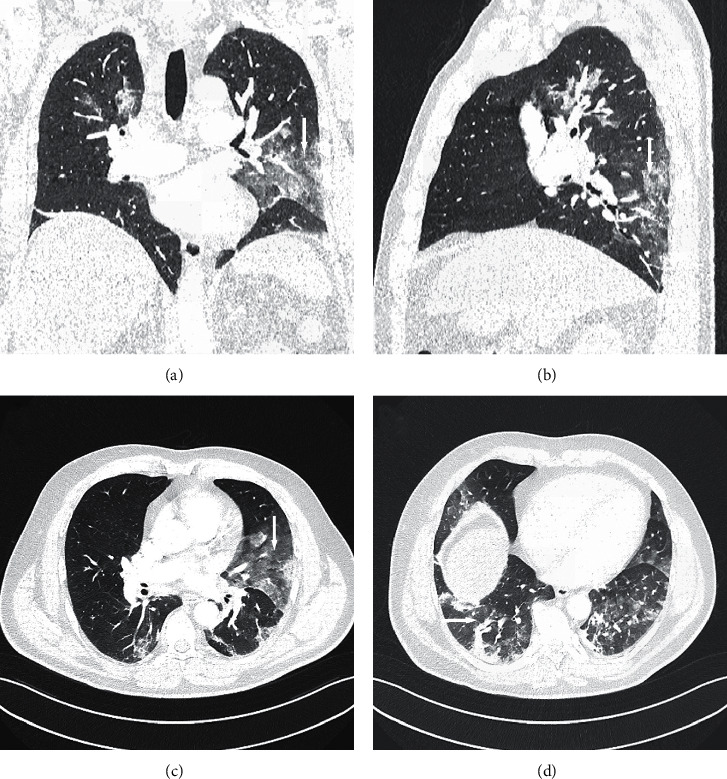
Chest computed tomography: (a) reconstruction in the coronal plane—“ground glass” opacities in both lungs; (b) reconstruction in the sagittal plane—“ground glass” opacities in the lower left lobe; (c) axial plane—“ground glass” opacities with a predominance of the left side; (d) axial plane—“ground glass” opacities and small areas of consolidation.
